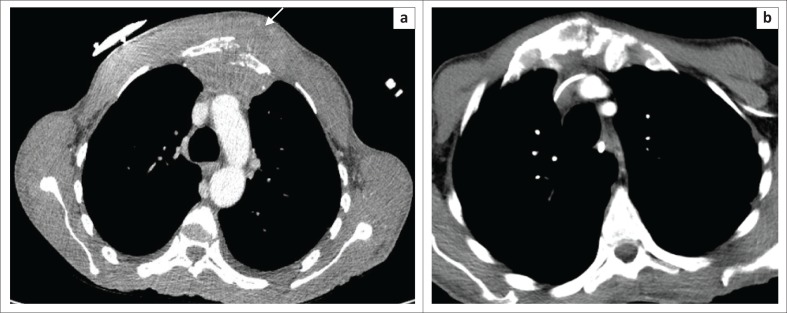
(a) Axial post-contrast CT imaging of the thorax on soft tissue windows demonstrates destruction of the sternum with surrounding soft tissue mass lesion (arrow); (b) Post-contrast imaging 1 month later demonstrates a good response to treatment, with marked reduction in soft tissue volume and bony infiltration.
